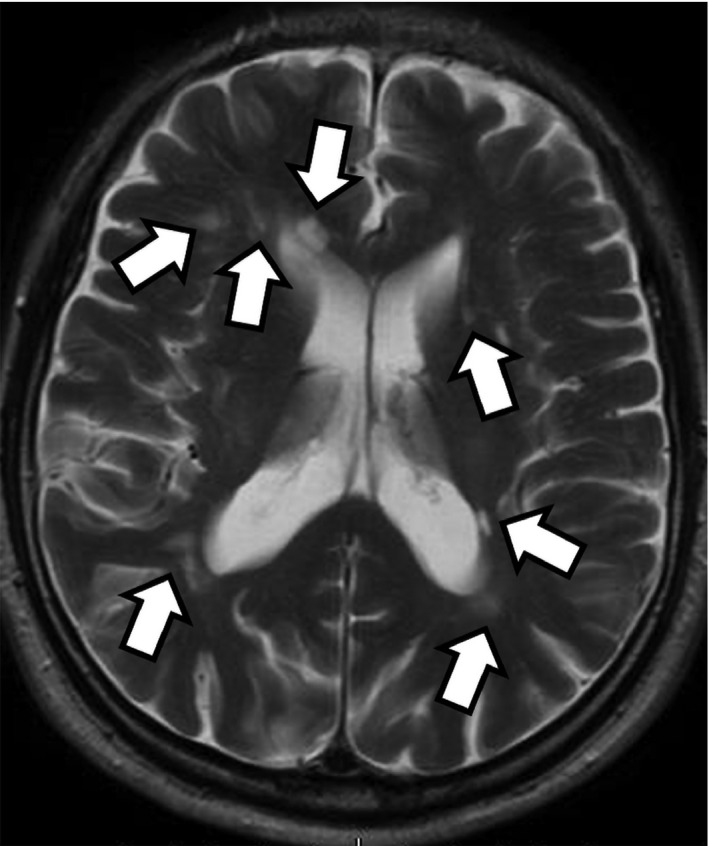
Brain MRI (T2‐weighted images) taken 6 months after discharge showed further improvement of multiple lesions in the cerebral white matter (arrows: CNS demyelination).
